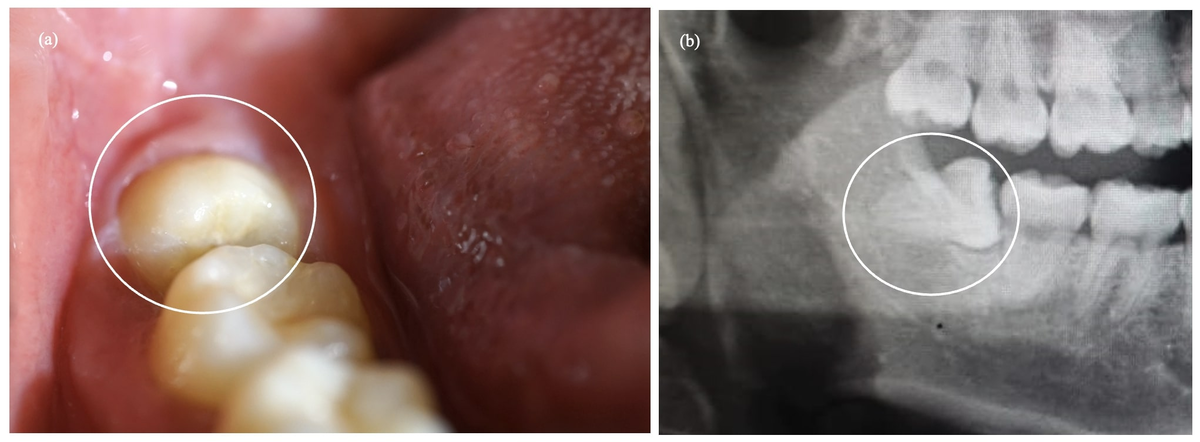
Preoperative image of the clinical and radiographic condition of the patients.
